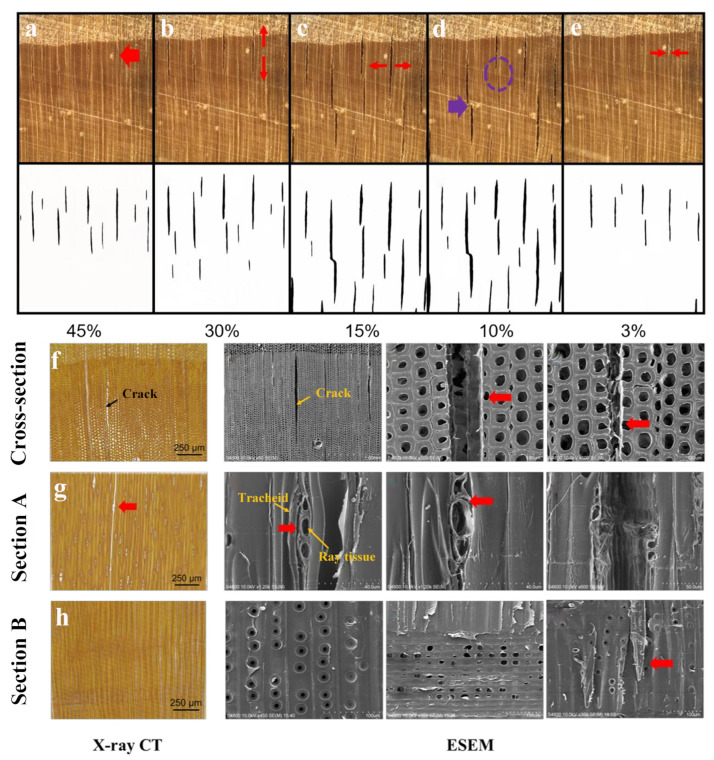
Generation and propagation of drying cracking. Macroscopic cracking at different moisture contents (a–e); fracture morphologies of samples: X-ray CT images and ESEM images in three anatomy directions (f–h) [22].
